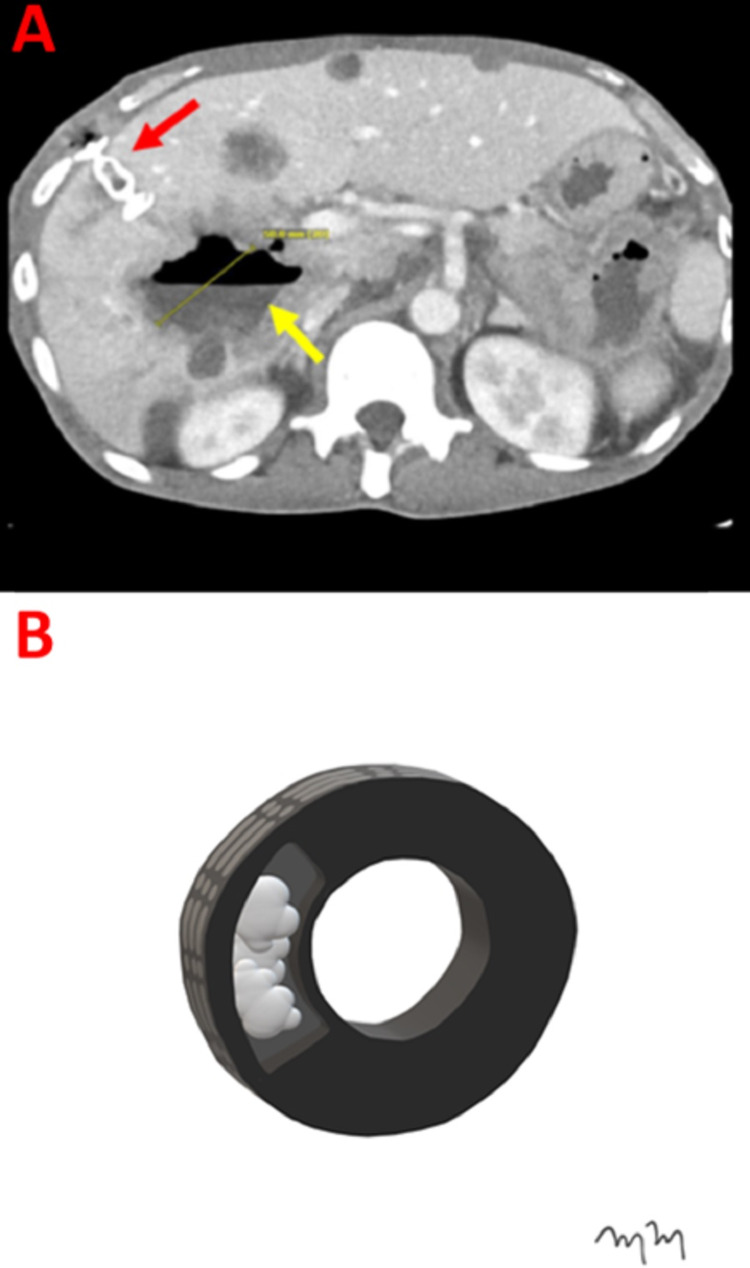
Artist Rendition of a Repaired Tire With Aerosol Tire Repair Analogous to Hemostatic Intrahepatic Abscess A post-procedural axial multiphasic computed tomography at the level of the inferior right hepatic lobe is shown (A). There is a significant interval decrease in size of the hemorrhagic intrahepatic abscess and expected air-fluid level within the mass (yellow arrow) related to the SURGIFLO® (Ethicon, Somerville, NJ) hemostatic matrix gelatin injection. There is no evidence of internal hemorrhage. The Amplatzer® vascular plug (St. Jude Medical, Plymouth, MN) within the previous percutaneous drain is in an unchanged position (red arrow) and without hemorrhagic extravasation. An artist rendition image (B) shows a functionally inflated tire with the aerosol tire repair matrix and complete repair of the air leak, analogous to the hemostatic intrahepatic abscess.
